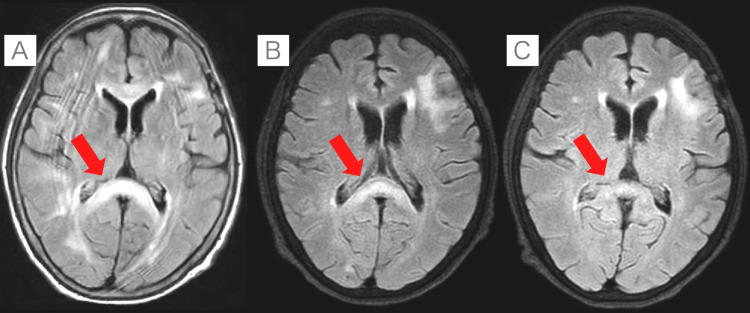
Temporal changes in MRI fluid-attenuated inversion recovery (FLAIR) images of metronidazole encephalopathy (red arrows) (A) MRI image showing a hyperintense lesion in the splenium of the corpus callosum consistent with metronidazole-induced encephalopathy. (B, C) Images taken on the 9th and 14th days after diagnosis, respectively. The hyperintense lesion in the splenium of the corpus callosum shows a gradual reduction over time.
